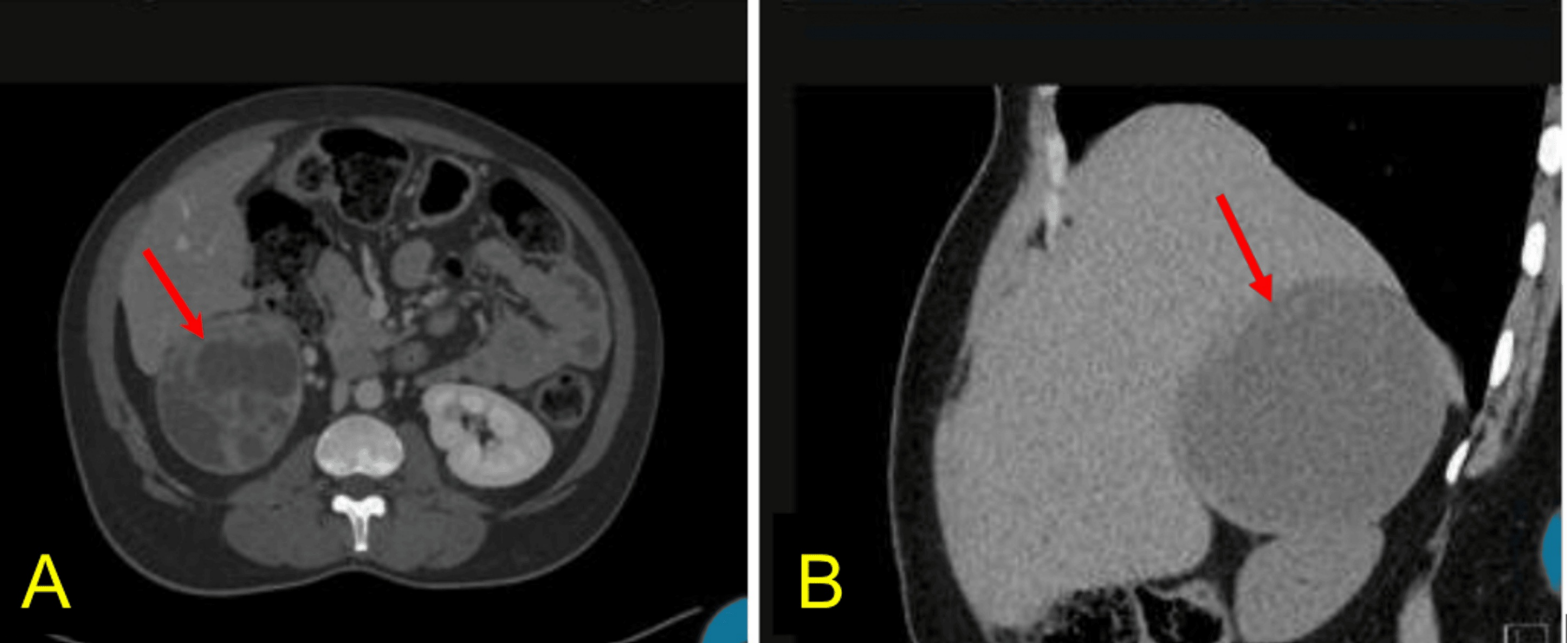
A: CT abdomen with contrast of a sagittal section of a focal soft tissue mass (red arrows) measuring 10.1 x 9.2 x 8.5 cm. The mass demonstrates predominantly low attenuation with internal thin septations without calcification. The margins are smooth, suggesting a non-infiltrative border. There is no capsular retraction or invasion of adjacent structures. B. A cross-sectional CT with contrast showing the lesion (red arrows) with enhancing thin septation, arterial enhancement with increasing enhancement on the venous, and delayed phases with no washout and an internal heterogeneous density with hyperdense foci, consistent with internal hemorrhage. There were no definite signs of vascular invasion or extrahepatic extension.
